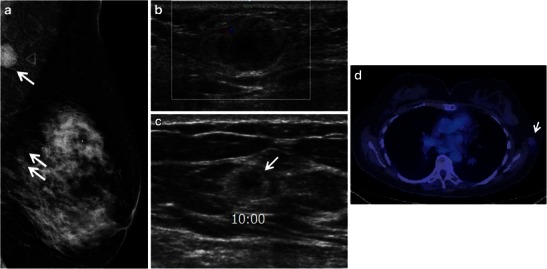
A 57-year-old woman with known multiple myeloma of the sacrum and multifocal involvement of the left breast and low axilla. a Full-field digital medio-lateral oblique view of the left breast demonstrates a round, circumscribed 1.9-cm mass (*arrow*) in the upper outer left breast/low axilla corresponding to a palpable abnormality. Two additional circumscribed masses were noted in the superior left breast posterior depth (*arrows*). b Ultrasound of the palpable low axillary mass imaging demonstrates a 2.0-cm heterogeneous mass with circumscribed margins with only mild increase in vascularity on colour Doppler. c Ultrasound of the mass at the 10 o’clock position of the left breast shows a microlobulated principally echogenic mass with central area of hypoechogenicity (*arrow*). The mass at the 9 o’clock position of the left breast had a similar sonographic appearance (*not shown*). Ultrasound guide core biopsy of all three lesions showed multiple myeloma. d Minimal FDG uptake is noted within the left upper outer/low axillary mass (*arrow*) on PET/CT
